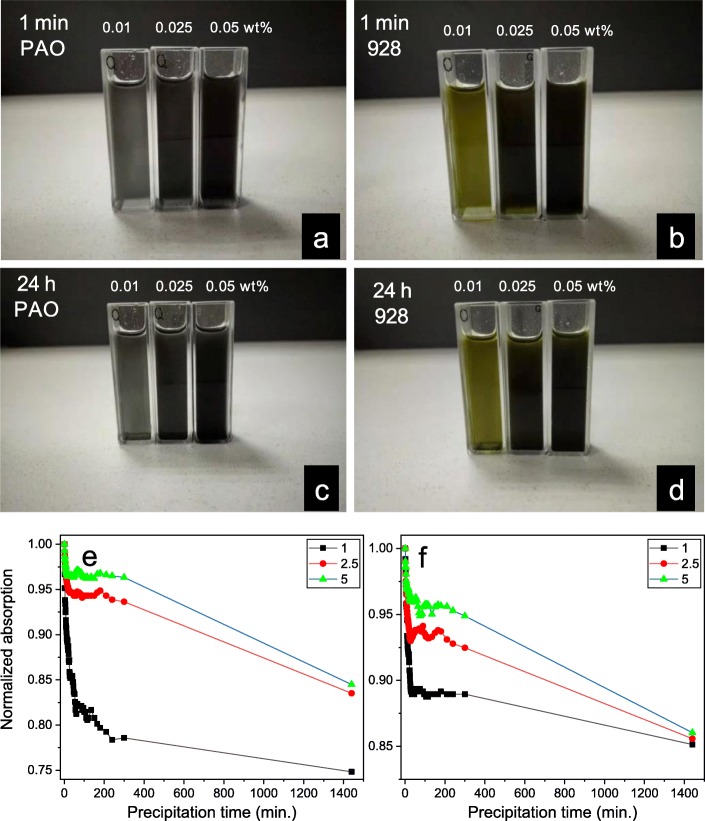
The optical photographs of the SA-GO (which is prepared by the reactions of octadecylamine and the sulfur-doped graphene oxide; the oxidation time of the graphene oxide is 24 h) ultrasonically dispersed in the PAO4 base oil (a) and the 928 aviation lubrication oil (b) and the PAO4 (c) and 928 (d) oil sample stand still for 24 h, respectively; the normalized adsorption of the SA-GO in PAO4 (e) and 928 (f) oil samples (a–d, examined by UV-vis spectrophotometer) with the concentration of SA-GO is 1, 2.5, and 5 × 10^−4^ wt% within 1440 min
